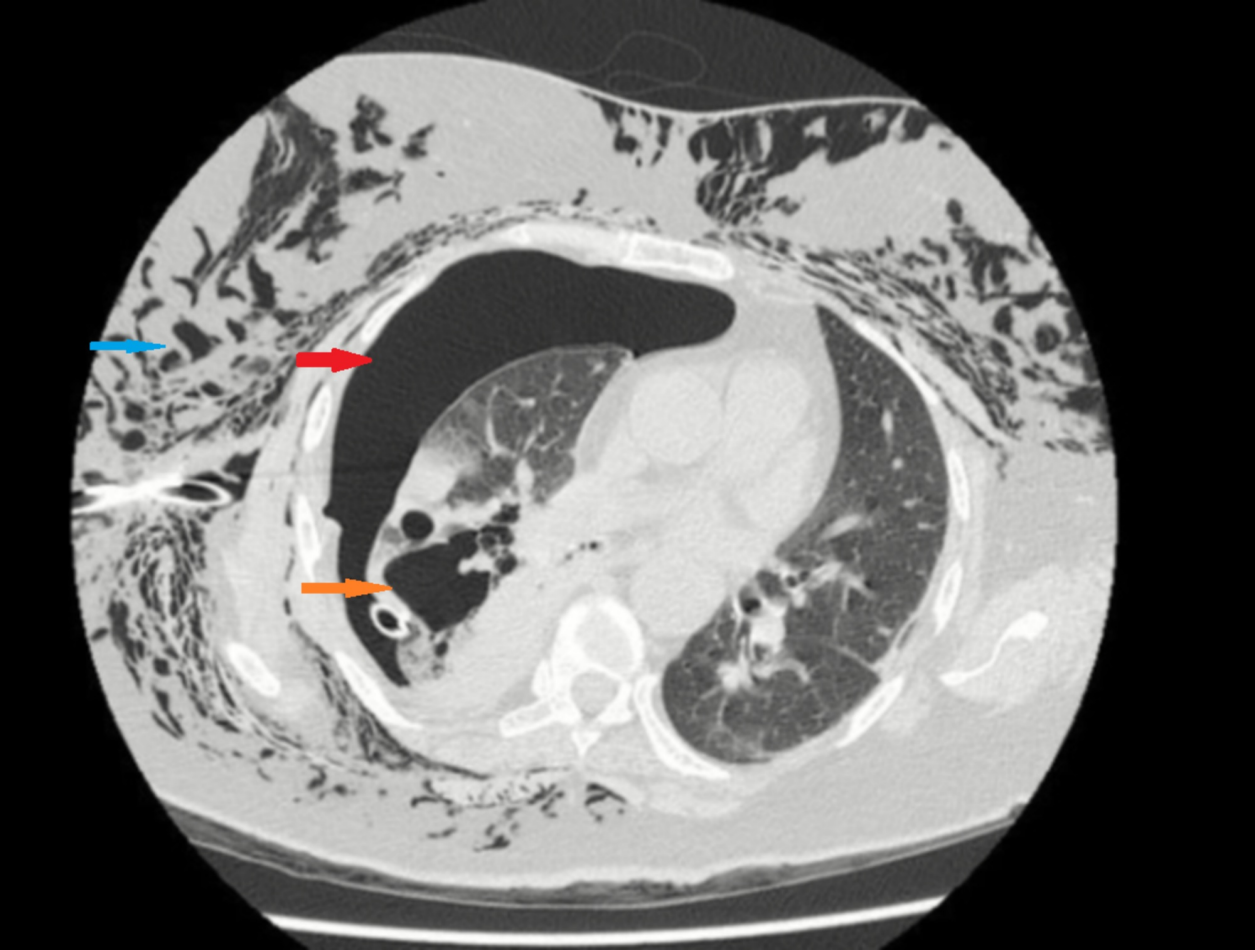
High resolution CT chest showing right cavitation (orange arrow) with pneumothorax (red arrow) and subcutaneous emphysema (blue arrow).
